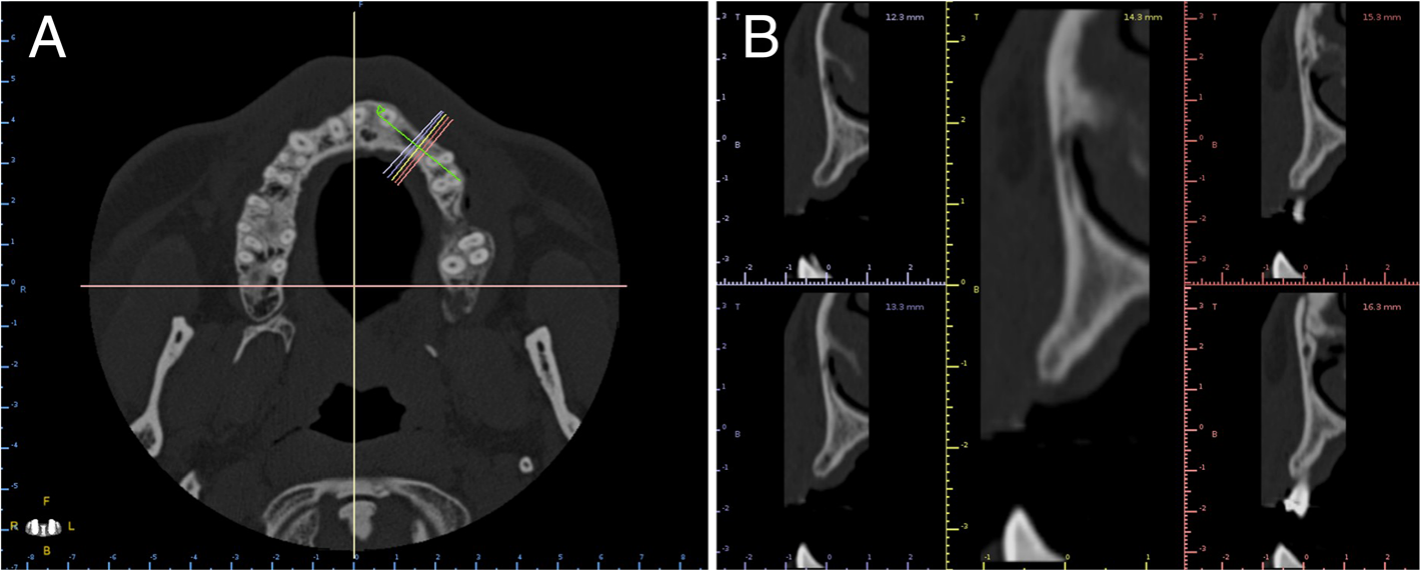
Preoperative anatomic situation on CBCT axial (a) and cross-sectional (b) images
